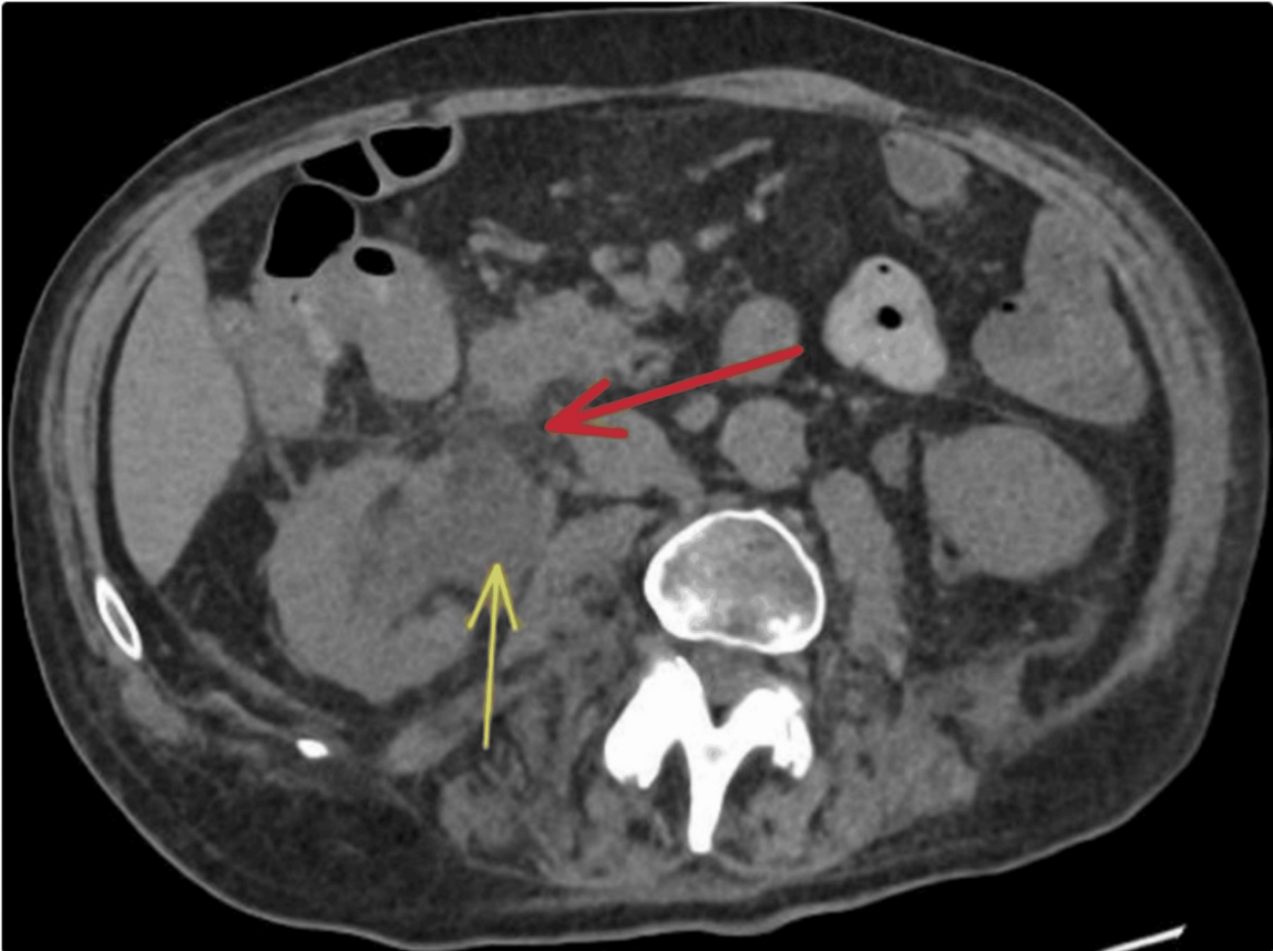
Axial view of the CT scan of the abdomen and pelvis. The red arrow points to the retroperitoneal fat stranding, and the yellow arrow points to the resulting right pelvicalyceal dilatation.
